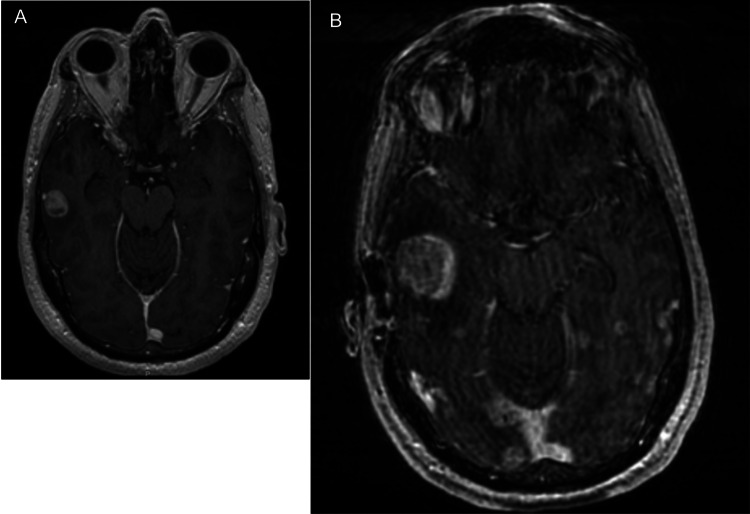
A: Patient’s T1-weighted MRI at the initiation of BRAF plus MEK inhibitors, showing a 1.5 cm brain lesion; B: Patient’s T1-weighted MRI six months after initiation of BRAF plus MEK inhibitors, showing the same lesion that now measures 3 cm The patient is symptomatic with seizures.
